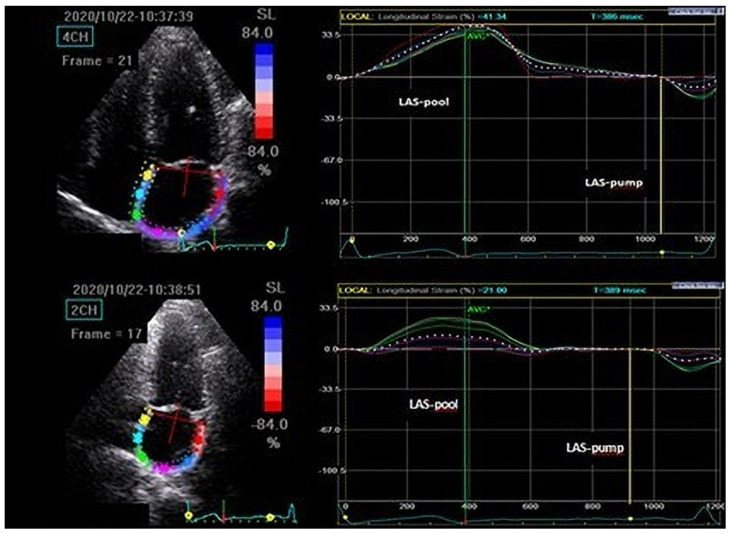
Left atrial two-dimensional speckle tracking echocardiography. Abbreviation: LAS, left atrium strain.
